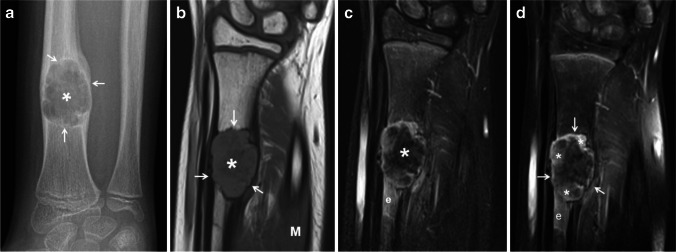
Solid variant aneurysmal bone cyst of the right radius in a 9-year-old girl. a An anteroposterior radiograph of the right wrist shows an expansile, lytic lesion (*asterisk*) involving the distal diaphysis of the radius. The lesion is geographical with endosteal scalloping and a sclerotic border (*arrows*). Minimal nonaggressive periosteal reaction is present proximally. b A coronal T1-weighted magnetic resonance (MR) image shows the lesion (*asterisk*) as solid and expansile with a slightly hyperintense parenchyma compared to muscle (*M*) and a sclerotic border (*arrows*). c A coronal fat-suppressed T2-weighted MR image shows the expansile lesion (*asterisk*) as heterogeneous in signal but predominantly hypointense. Note the adjacent bone marrow edema (*e*). d A coronal fat-suppressed, contrast-enhanced, T1-weighted MR image shows patchy, heterogeneous contrast enhancement (*asterisks*) by the lesion (*arrows*) and by the adjacent bone marrow edema (*e*)
